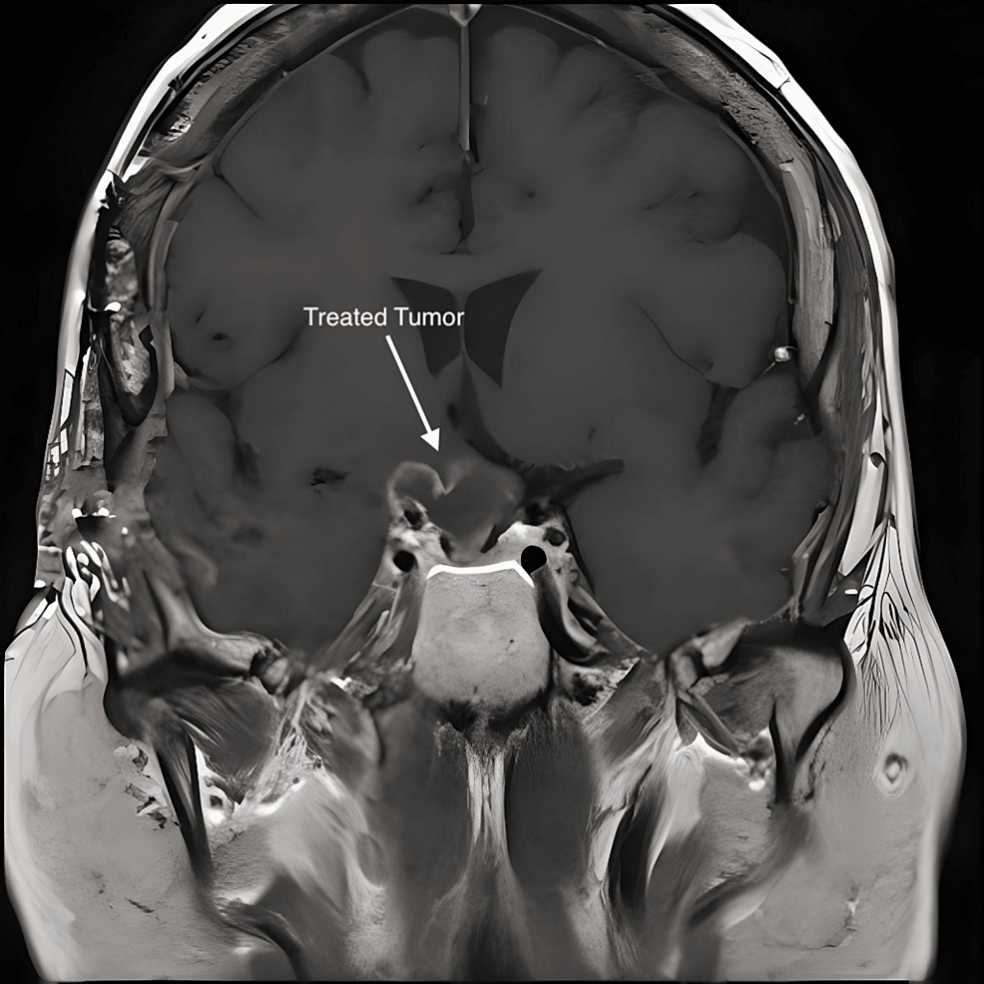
Mid-coronal MRI with contrast; taken after the debulking procedure, the scan demonstrates persistent cystic compression of the chiasm and the right optic nerve
